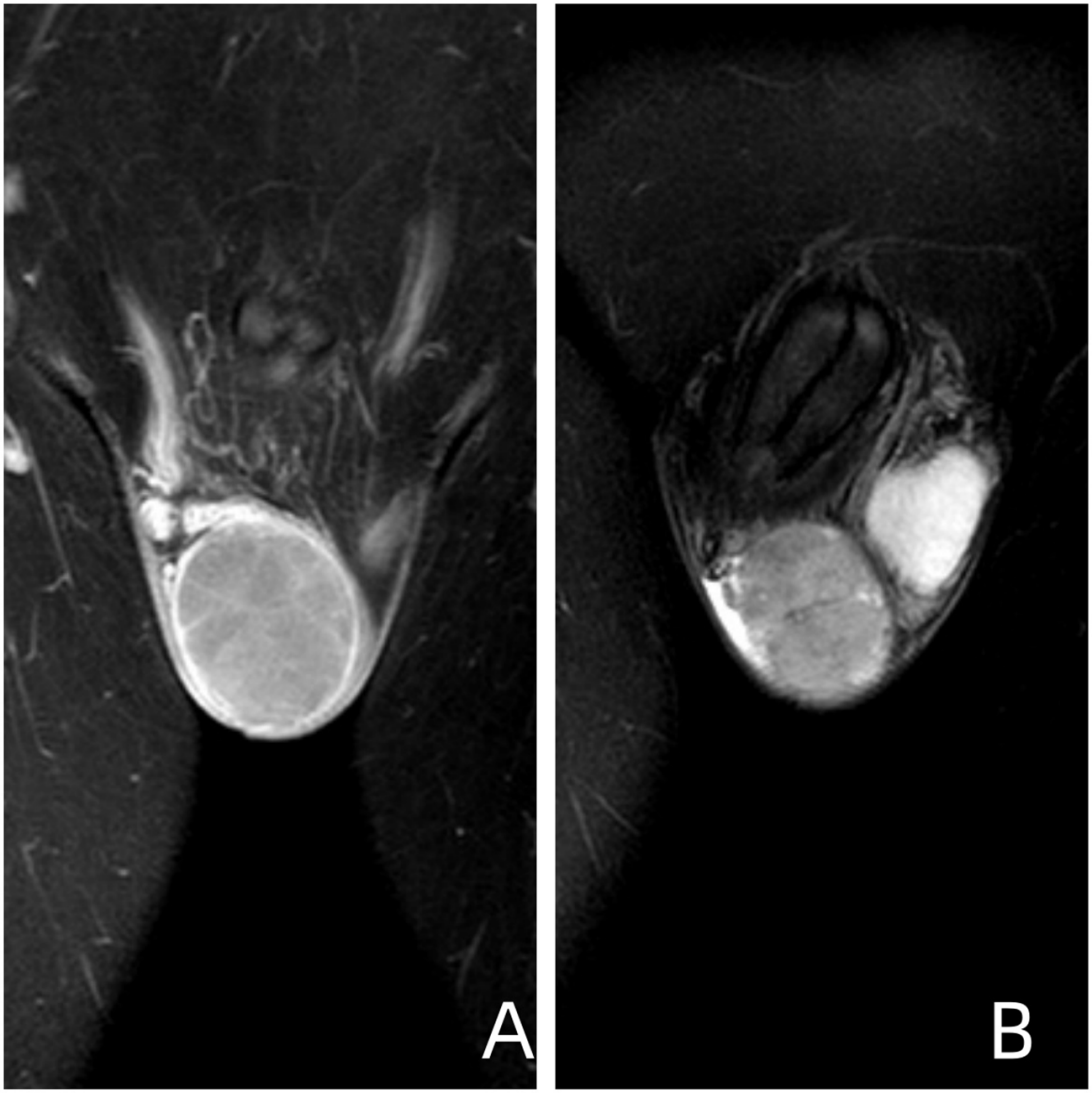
Image Descriptions and Findings (A) Coronal T1-weighted image of the testis obtained using contrast-enhanced multi-slice spiral CT. (B) Coronal T2-weighted fat-suppressed image of the testis. Imaging Conclusion: The right testis exhibits a roughly spherical mass measuring approximately 47mm x 52mm x 53mm. The findings are suggestive of embryonal carcinoma.
